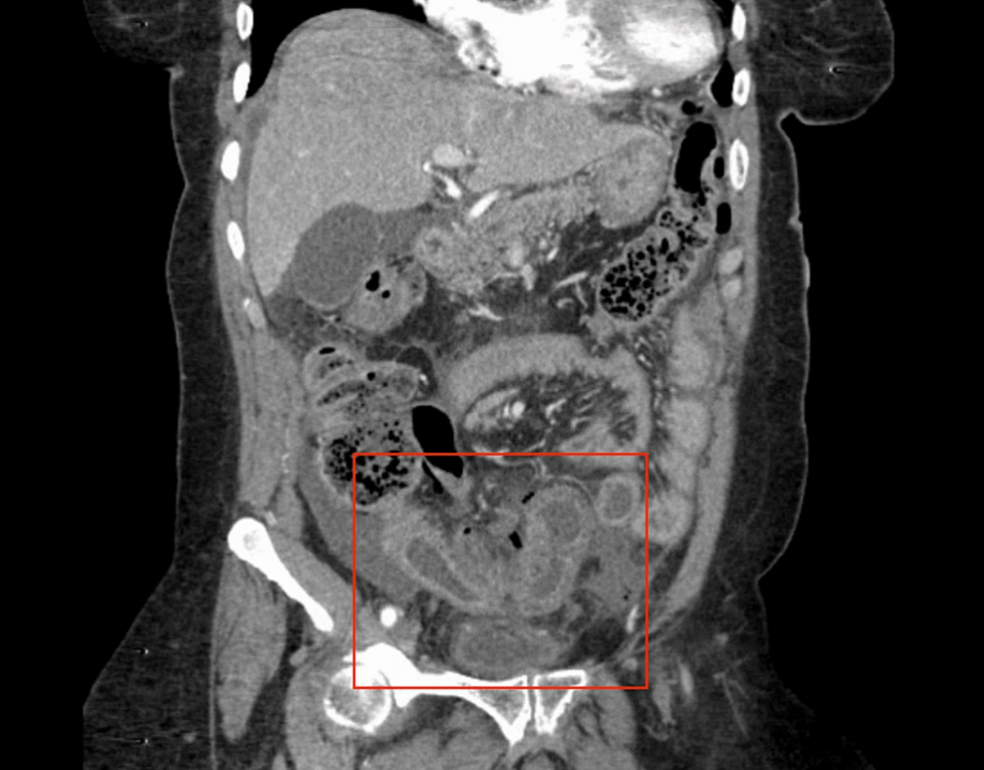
CT Angiography/Abdomen and Pelvis With and Without Contrast Coronal View The abdomen and pelvis CT scan revealed an inflammatory adhesion between the small bowel and sigmoid colon (red box).
